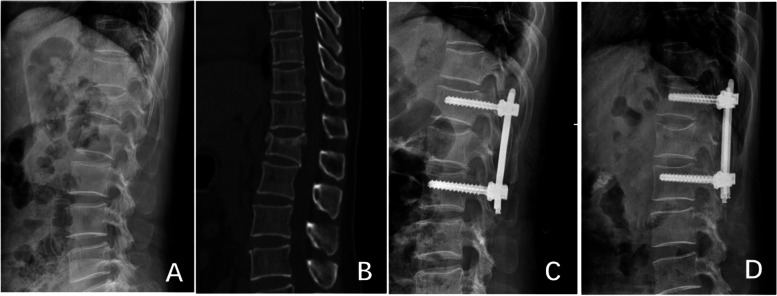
A 52-year-old female was diagnosed L1 vertebral fracture (AO type A1) by DR and CT (A, B). Percutaneous short segment pedicle screw fixation without screwing of the fractured vertebra with O-arm navigation was operated (C), and he got the satisfactory recovery (D)
